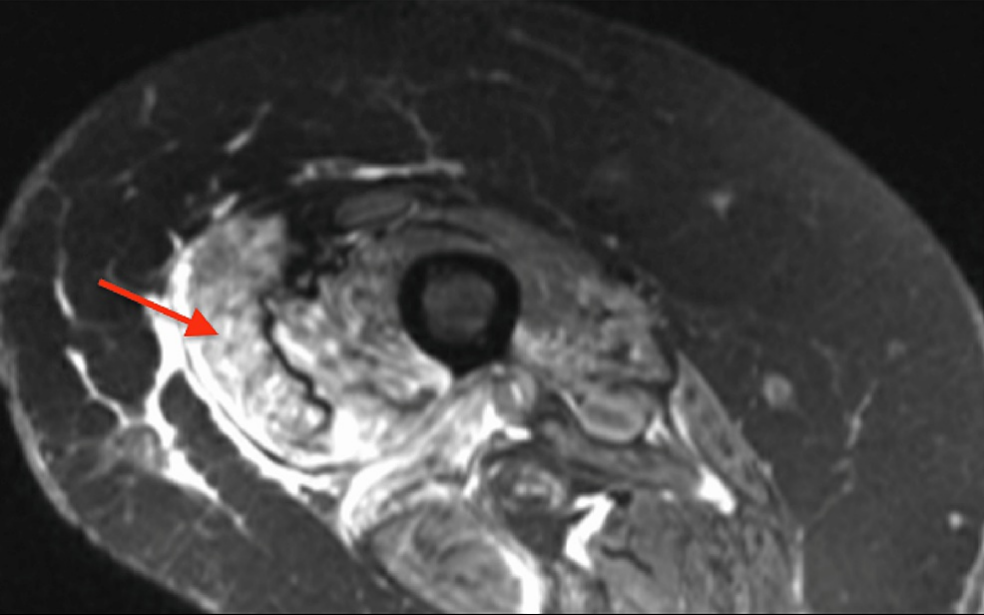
Magnetic resonance imaging with increased T2 signal intensity on the thigh musculature as indicated by the arrow
